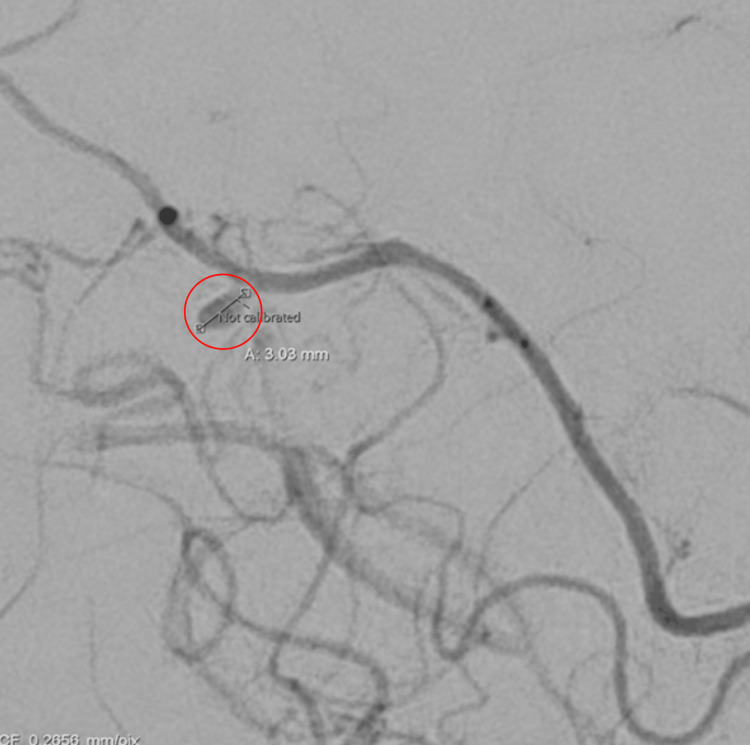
Repeat CT angiography of the head with contrast showing an associated 0.3 cm aneurysm (red circle) involving the distal right anterior cerebral artery.
